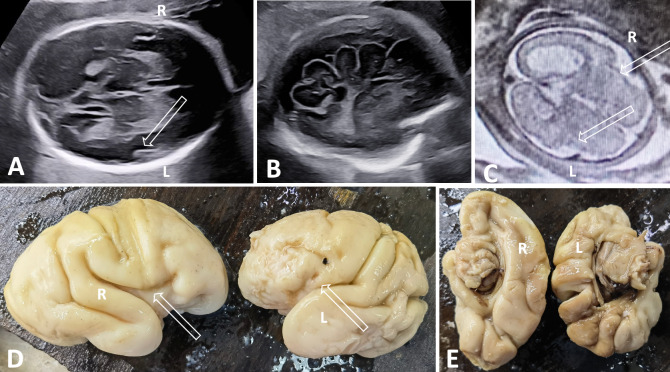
Patient 6, the 24.6-gestational-week-old fetus of a 34-year-old woman, had megalencephaly–polymicrogyria (L) and ventriculomegaly (R). A Axial plane showing irregular SF without a plateau-like insula (hollow arrow). B Parasagittal plane showing abnormal sulci in the left hemisphere with a seesaw appearance. C Axial MR image showing hemisphere asymmetry, abnormal SF (hollow arrow, L) and ventriculomegaly (R). D Comparison of lateral surfaces of the brain by postmortem examination showing abnormal SF (hollow arrow, L) and polymicrogyria in the frontal lobe (L, arrows). E Comparison of the medial surfaces of the brain by postmortem examination revealed that the left hemisphere was smaller with abnormal sulci
